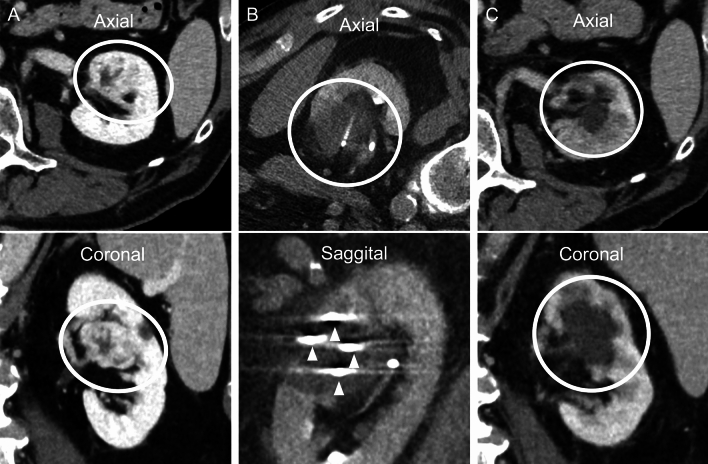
CT images from a 62-year-old male patient with a 38-mm endophytic biopsy-proven clear cell RCC in the left kidney where PCA was performed. A Preprocedural CT images with intravenous contrast, venous phase. The tumor is marked with a white circle. B Intraprocedural CT images with the patient in an oblique position. The image demonstrates the iceball zone. C Venous contrast CT imaging one year after primary PCA shows no residual tumor mass. The ablation zone is marked with a white circle. ^a^Computed tomography (CT). ^b^Renal cell carcinoma (RCC).^c^Percutaneous cryoablation (PCA)
